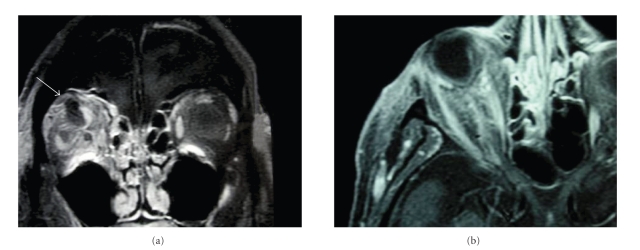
Cephalic and orbital MRI. (a) The arrow shows a hyposignal corresponding to the abscess, (b) exophthalmos stage I.
